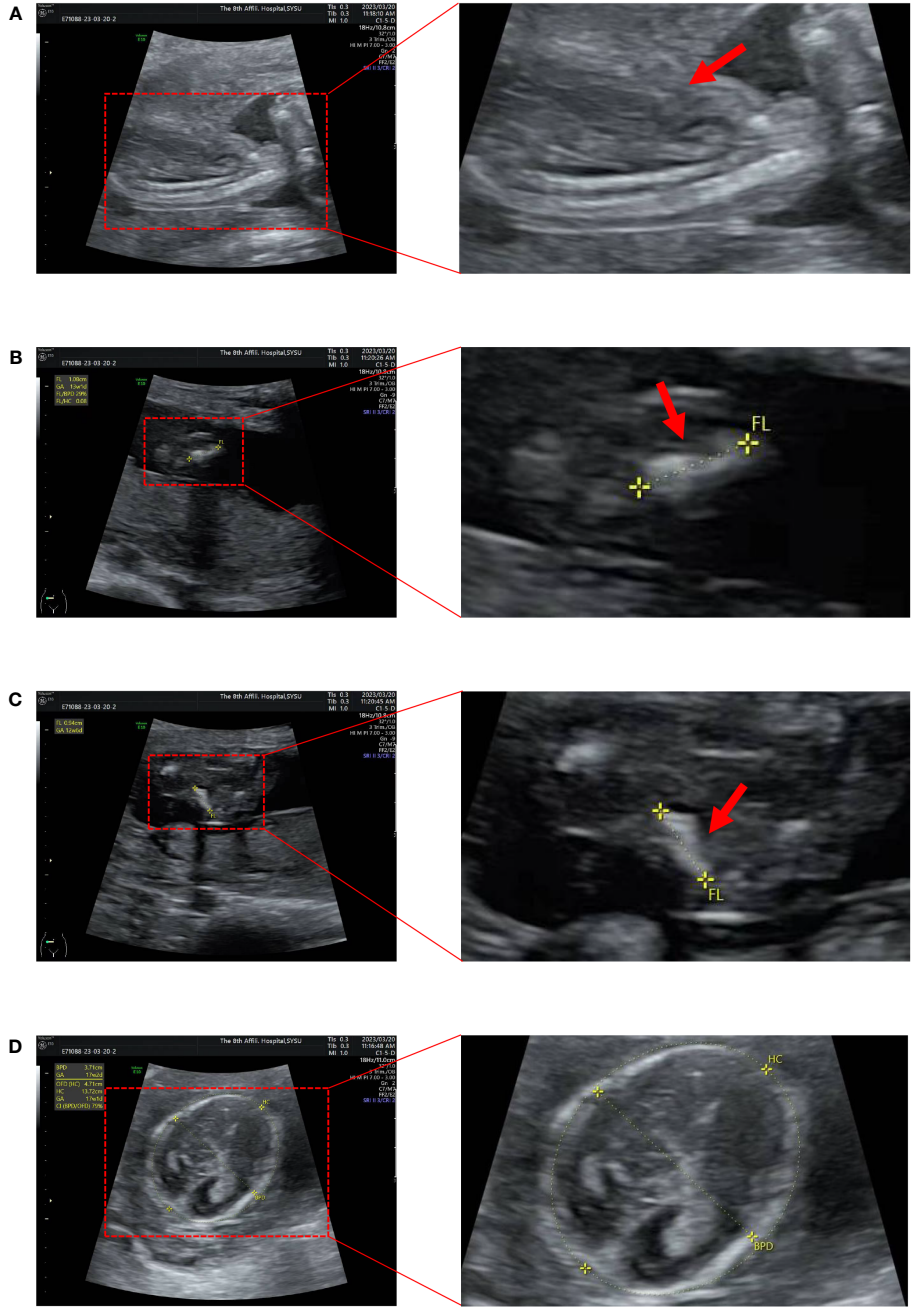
Ultrasound images of the fetus. (A) The thorax is narrowed and the notching can be seen at the thoracic-abdominal transition. (B, C) The femur is short and curved, in the shape of an earpiece. (D) Measure BPD and HC (BPD 37mm; HC 132mm).
